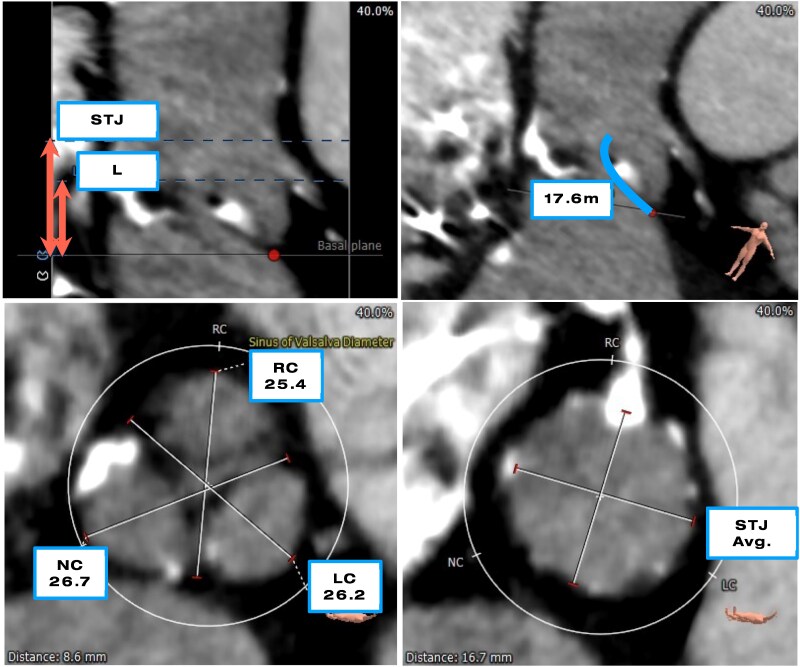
Pre-procedural planning with multi-slice computed tomography demonstrating considerable risk of left coronary artery obstruction with low coronary ostium height, 11.0 mm of left coronary artery (*A*). Calcified left coronary leaflet and leaflet length 17.6 mm (*B*) and shallow sinuses of Valsalva with diameter 26.2 mm at left cuspid (*C*). Sinotubular junction 22.3 mm in average diameter (*D*).
